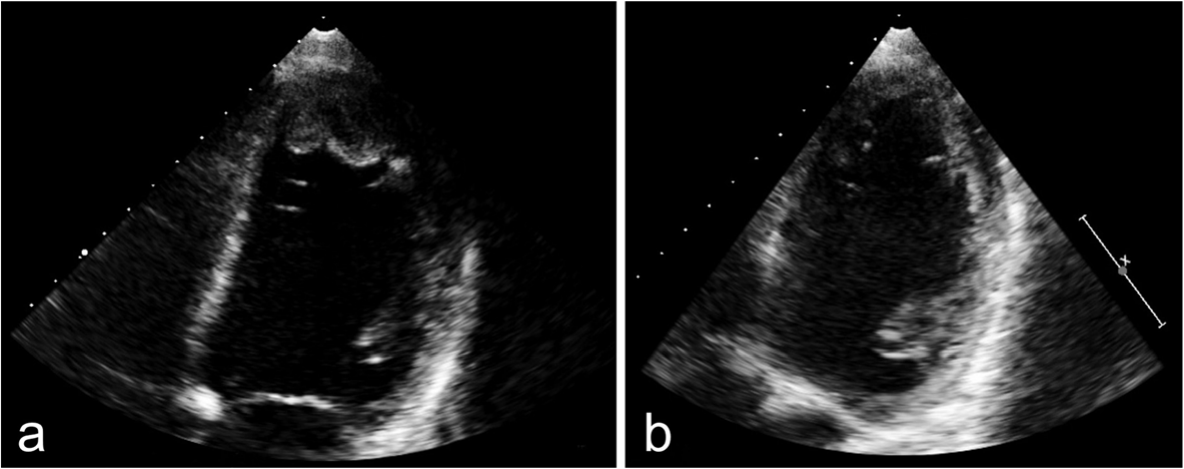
Echocardiography of the left ventricle shows thrombus formation in the apex (a). Following thrombolytic therapy with enoxaparin, echocardiography revealed complete disappearance of the thrombus (b).
